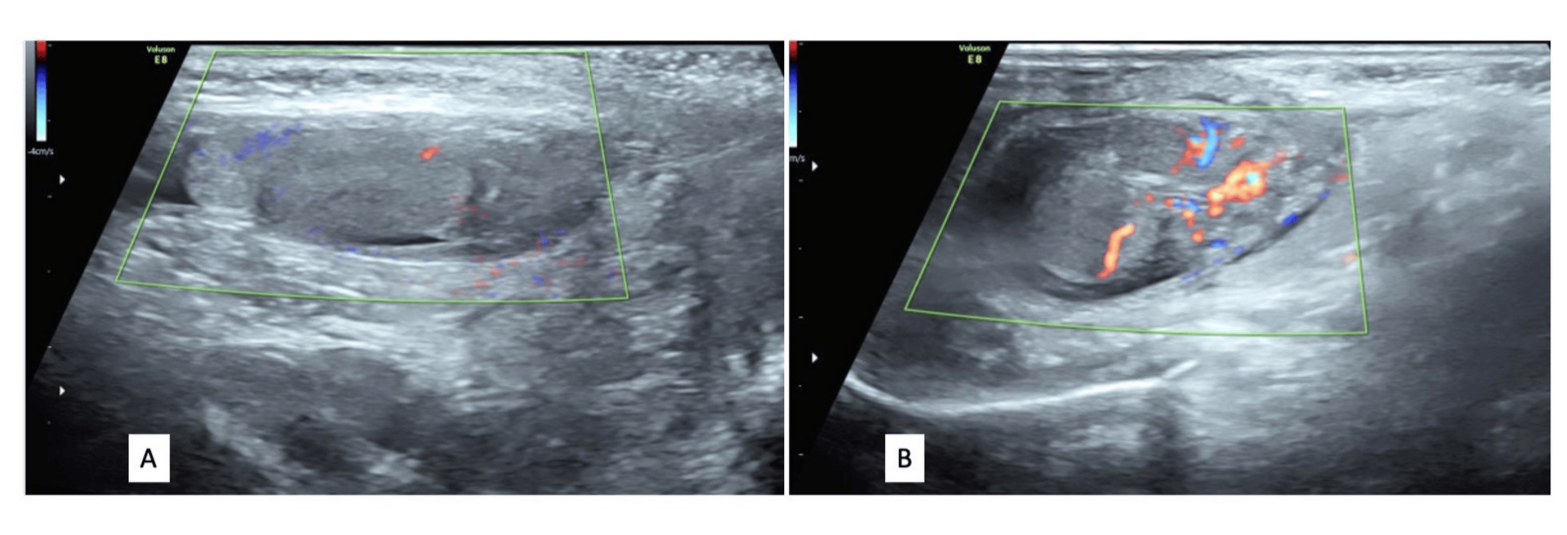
Color doppler ultrasound images of the right (A) and left (B) scrotal sacs showing increased vascularity of the left epididymis and testis
